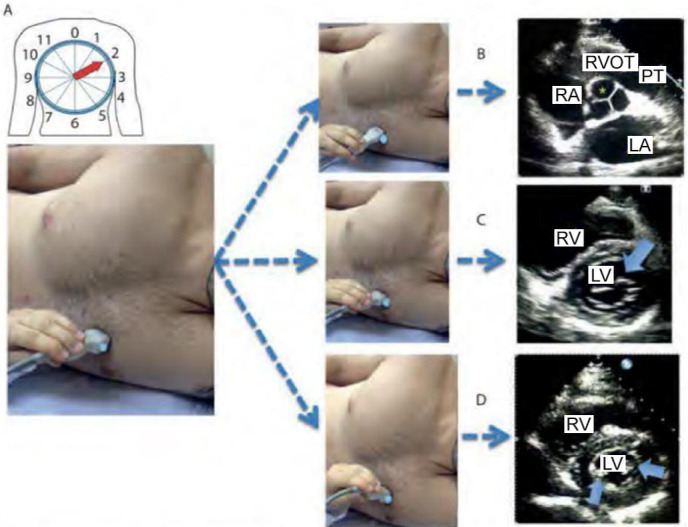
Several observation planes in the transverse parasternal window. (A)
